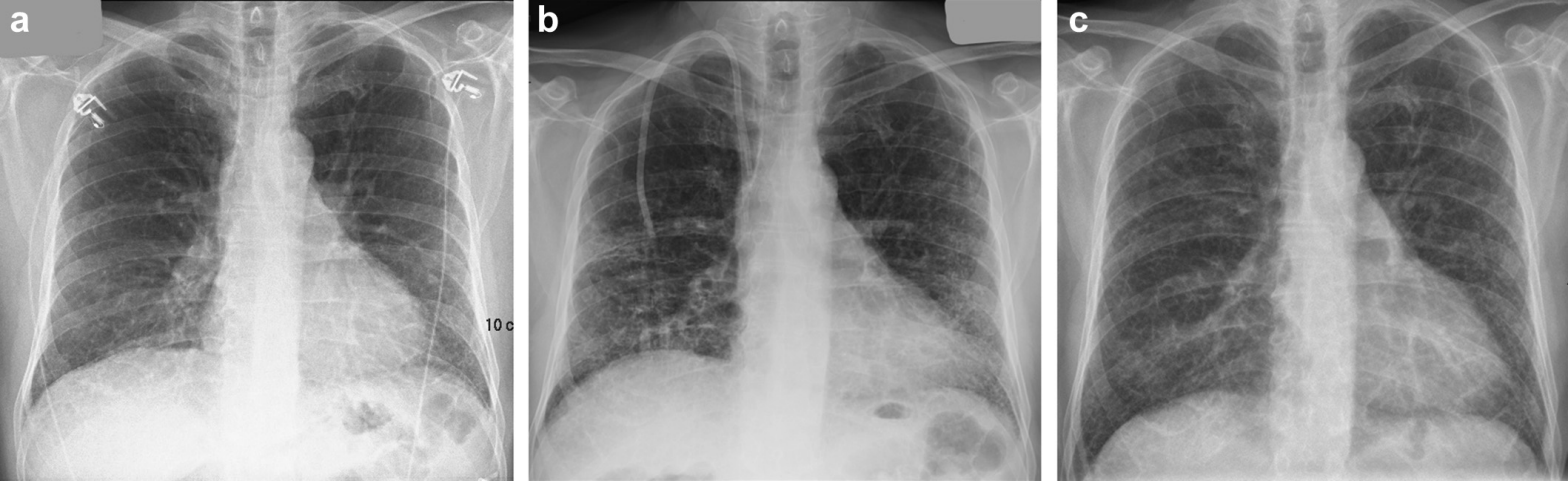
(a) X-ray taken before cyclophosphamide treatment. (b) X-ray taken during treatment. (c) X-ray taken 2 months after cyclophosphamide was discontinued. The pretreatment X-ray was reported as showing a largely peripheral and basal pattern of fibrosis, which then improved significantly following drug withdrawal.
